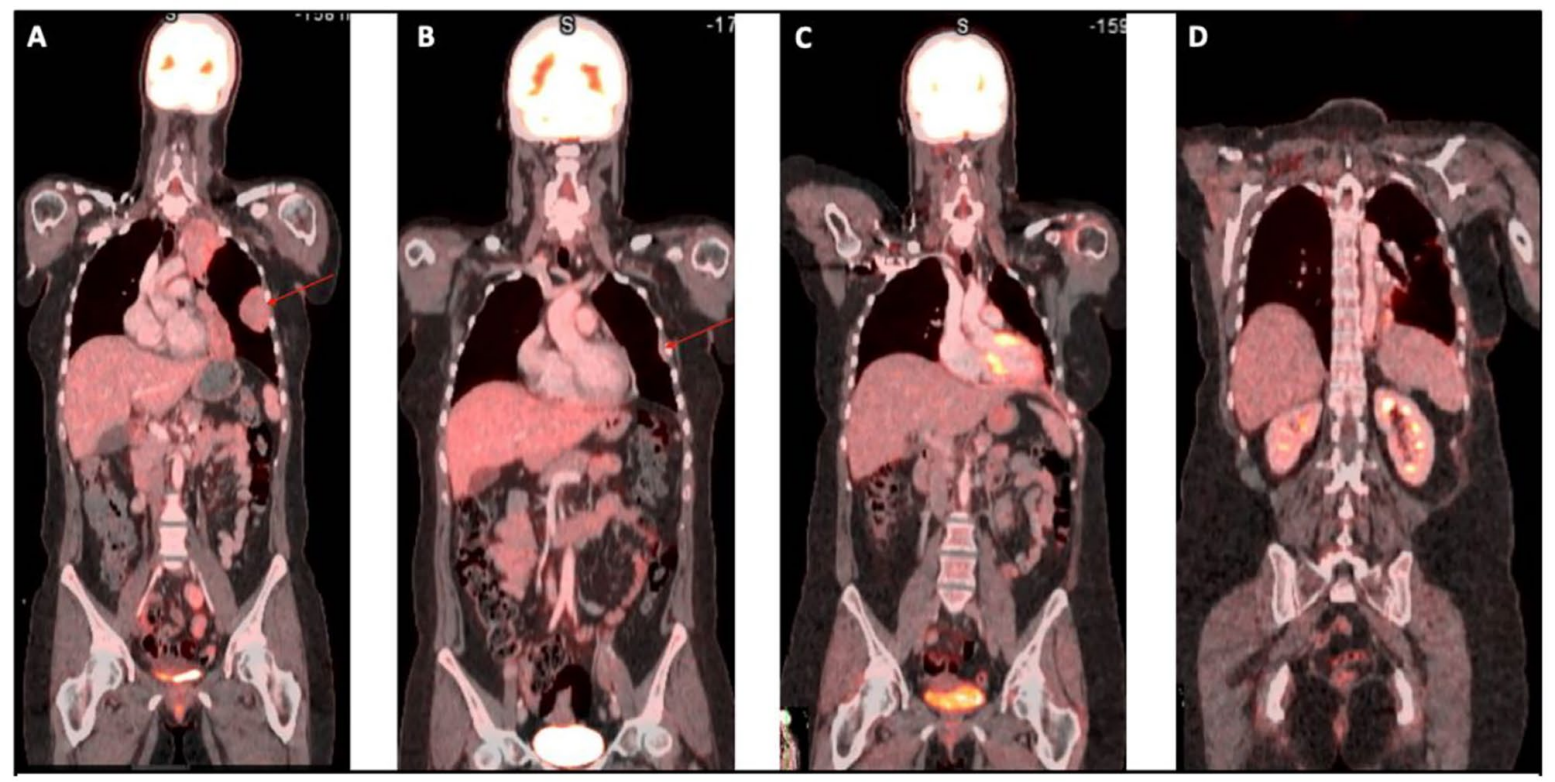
(A) PET-CT prior to chemotherapy; (B) PET-CT after neoadjuvant chemotherapy; (C-D) PET-CT 6 months after surgery
